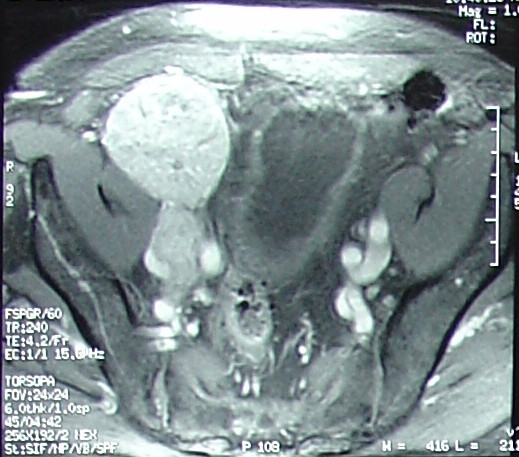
MRI of pelvis showing right lower quadrant mass compressing external iliac vessels.
